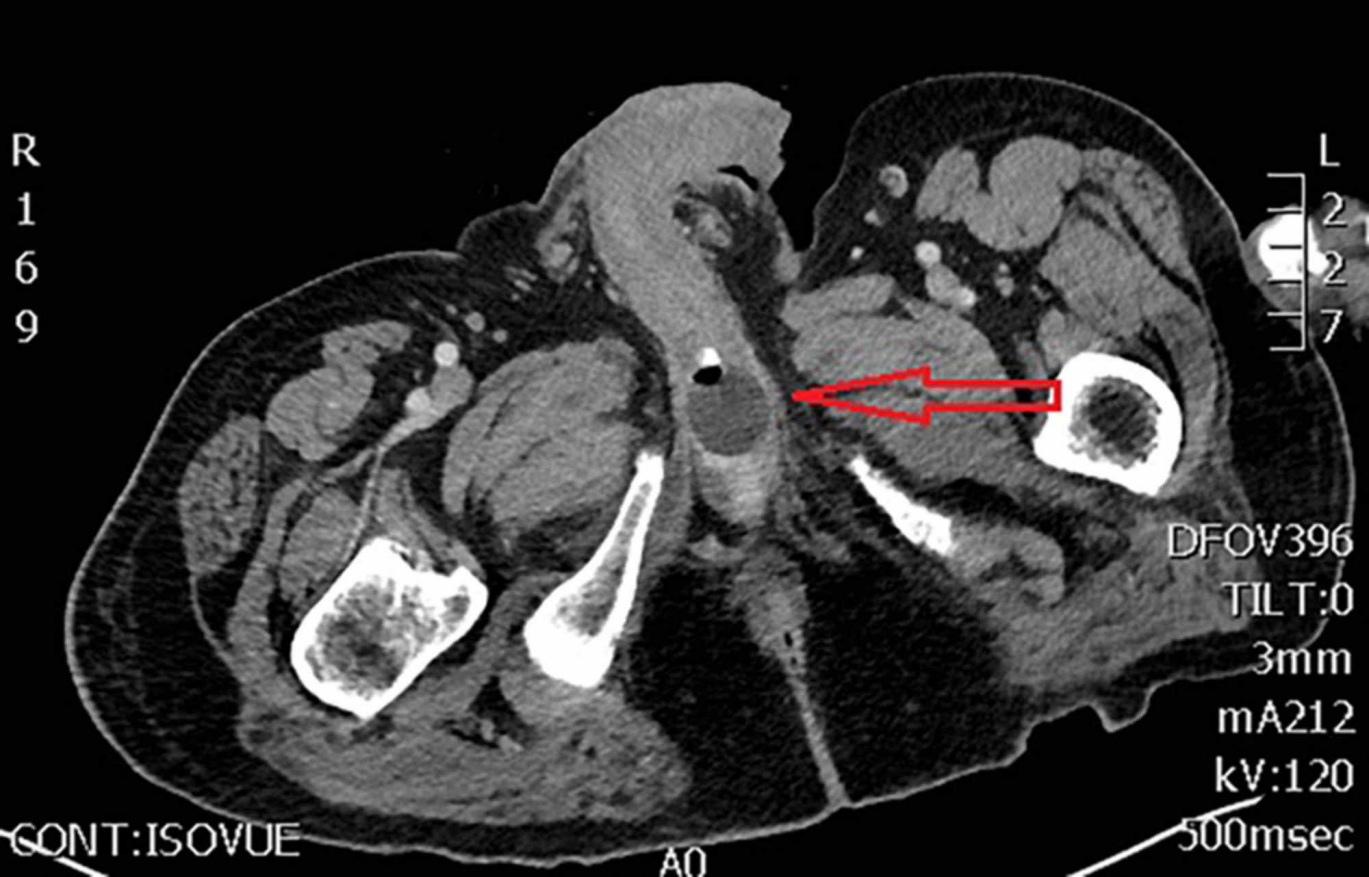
Axial CT scan with the arrow indicating inflated catheter in the penile urethra.
